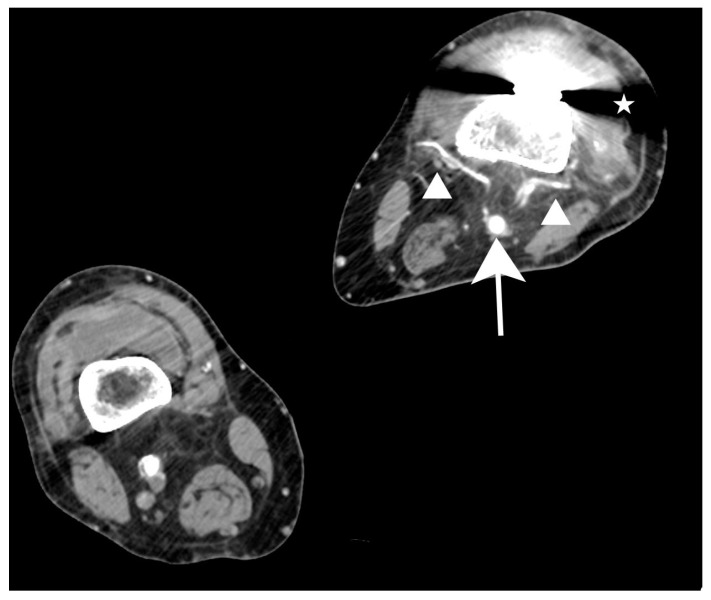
Representative CTA image of the knee joints after dislocation of the left joint—performed on the day of readmission to the hospital. Adequate contrast opacification of the popliteal artery (white arrow) and the arterial branches forming the genicular anastomoses (white arrowheads) is visible. Artifacts from the knee prosthesis (white asterisk) limit the assessment, but no contrast leakage outside the vessels was observed.
